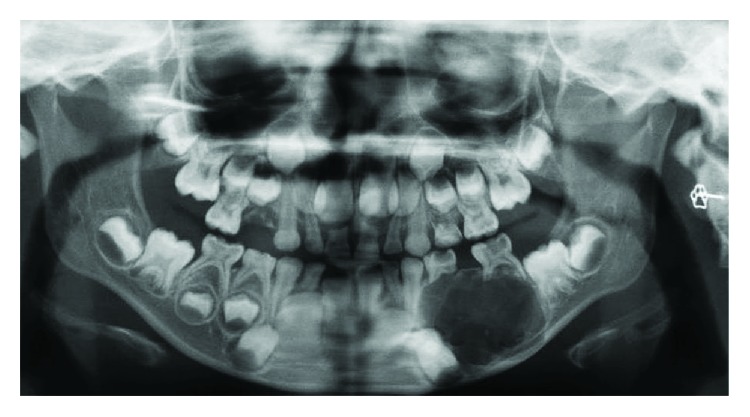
Orthopantomogram showing a well-defined multilocular radiolucent lesion with a sclerotic border in the left body of the mandible. The second deciduous molar has been displaced distally and is supraerupted.
